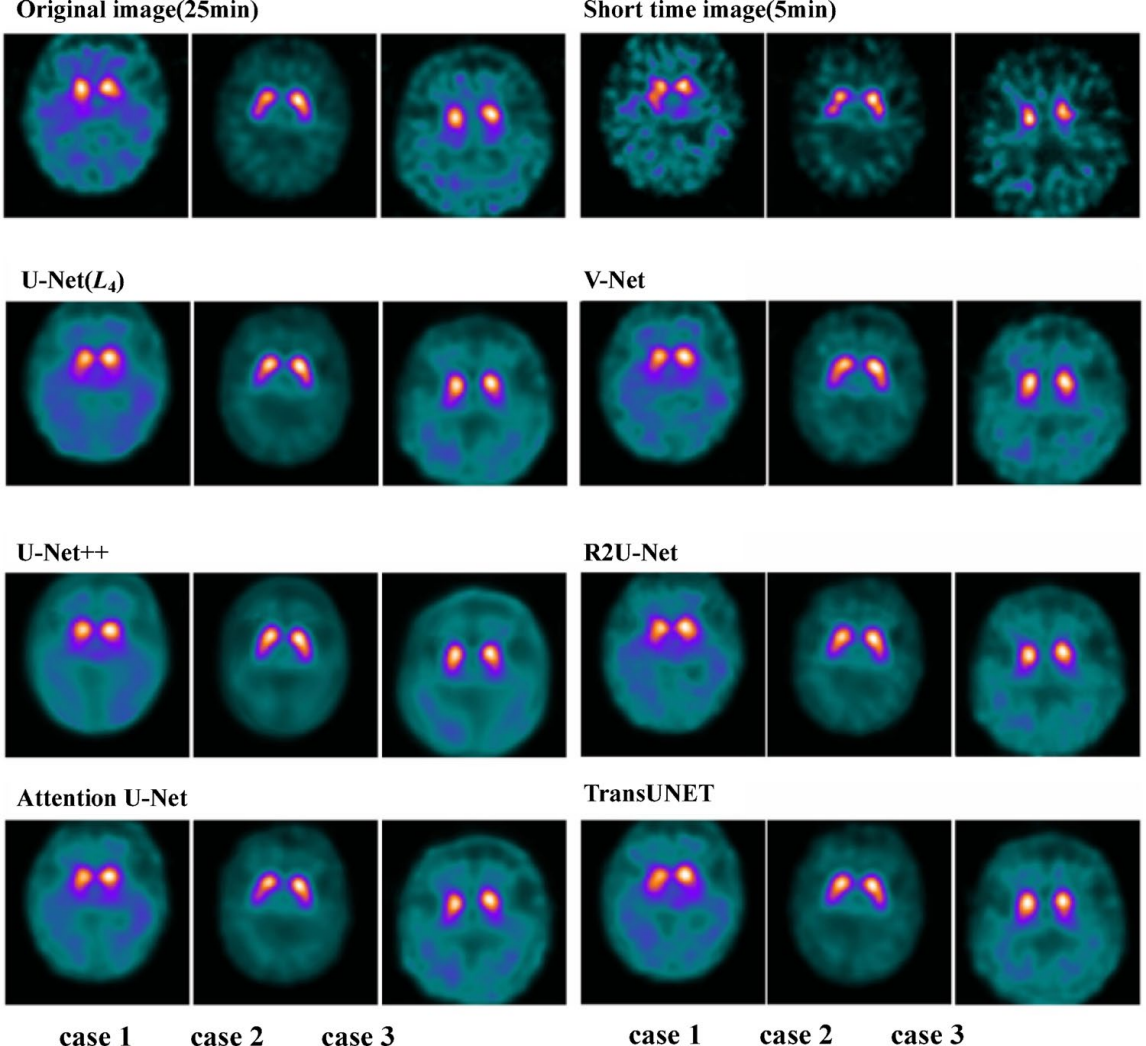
Qualitative performance of deep-learning reconstructions for short-time ^123^I-ioflupane SPECT. For three representative patients (columns), axial slices are shown for the 25-min reference image, the 5-min short-time image, and six deep-learning reconstructions (U-Net [*L*₄], V-Net, U-Net +  + , R2U-Net, Attention U-Net, and TransUNET). All panels share an identical color scale and intensity window. Deep-learning methods markedly sharpen striatal borders and reduce background noise when compared with the 5-min baseline, with the four-layer U-Net appearing most similar to the 25-min reference. *U-Net* U-Net (encoder–decoder CNN), *U-Net* +  + Nested U-Net, *V-Net* V-Net (3-D CNN), *R2U-Net* Recurrent residual U-Net, *Attention U-Net* Attention-gated U-Net, *TransUNet* Transformer-based U-Net
